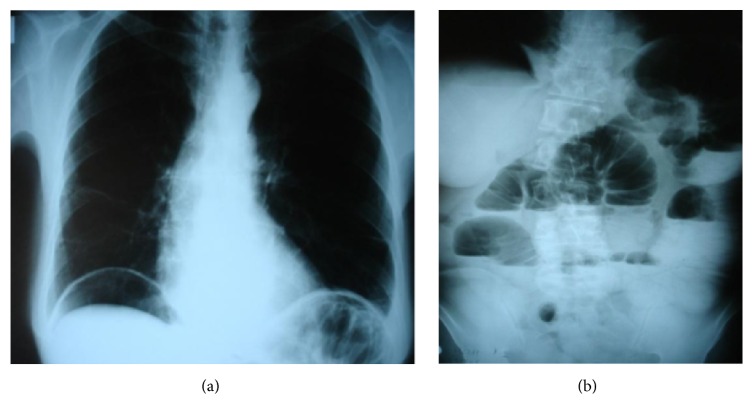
Abdominal X-ray after colonoscopy and clinical deterioration. Presence of free abdominal gas.
